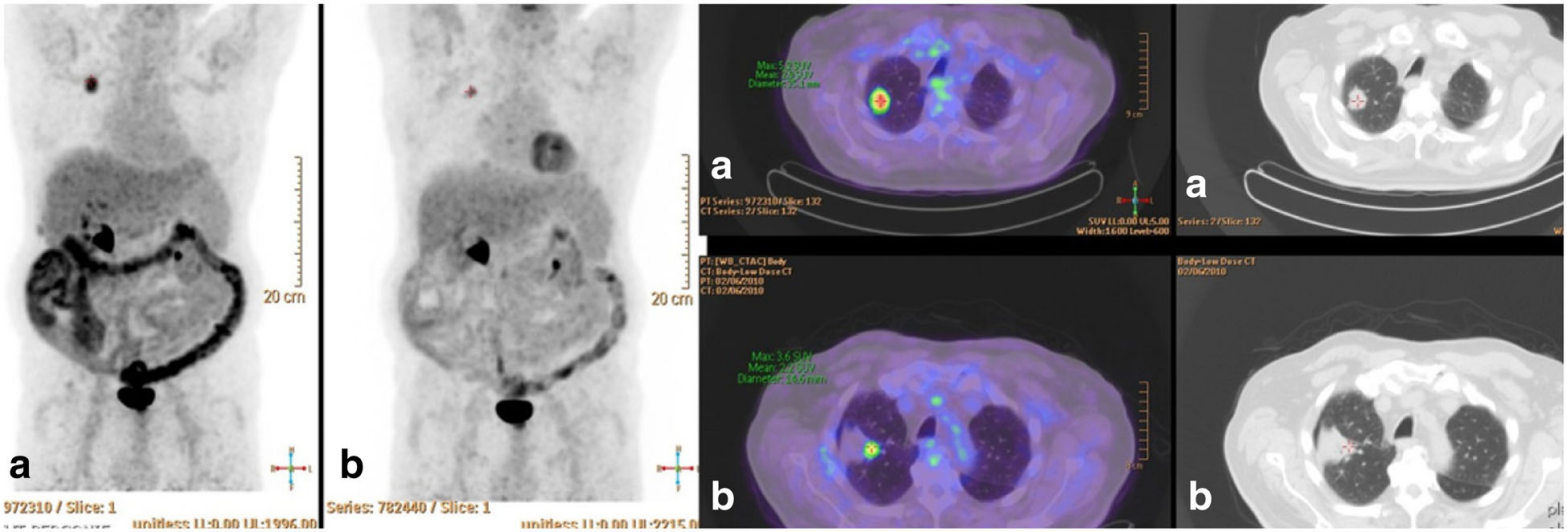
PET-CT before (a) and after RFA (b): an incomplete treatment can be seen on the edge of the ablation zone
